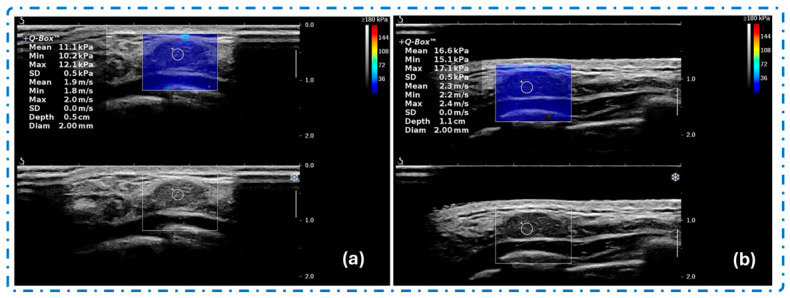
Comparative shear-wave elastography (SWE) ultrasound images demonstrating the effects of different acoustic coupling conditions on superficial tissue imaging. (a) SWE image acquired using conventional coupling conditions showing reduced uniformity and potential near-field artifacts. (b) SWE image acquired under improved coupling conditions demonstrating enhanced image clarity and more consistent acoustic coupling. Images in (a,b) are reprinted from Taylor & Francis under the Creative Commons Attribution 4.0 license [71].
